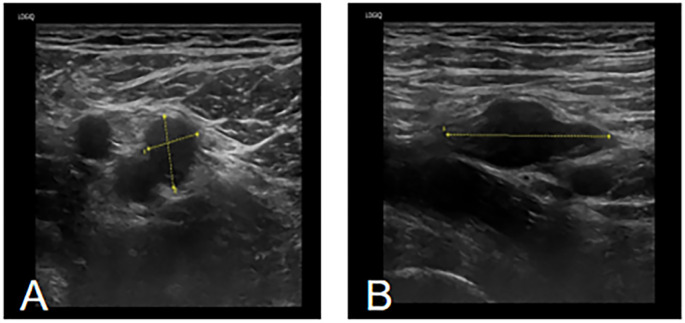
Ultrasound findings of the left thigh muscles and inguinal region. (A) Transverse grayscale ultrasound image showing a well-defined, homogeneously hypoechoic lesion adjacent to the left femoral artery. (B) Longitudinal grayscale ultrasound image of the same lesion demonstrating its configuration along the femoral nerve. No significant internal blood flow was detected on CDFI. Mild atrophy of the left thigh muscles was observed compared with the contralateral side. These findings are consistent with a probable lesion of femoral nerve origin, most likely a neurofibroma.
